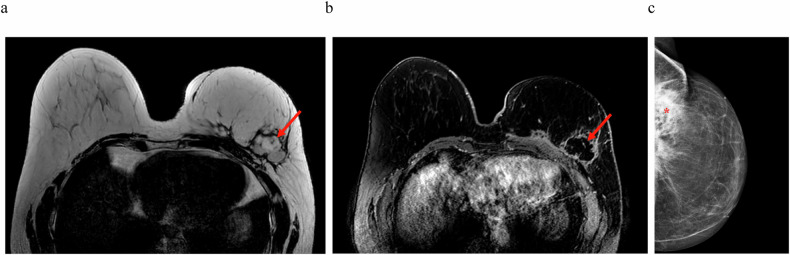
Fat necrosis after DIEP-flap breast reconstruction. A 67-year-old patient with a history of mastectomy because of DCIS underwent DIEP-flap reconstruction. Eight months after the reconstruction, fat necrosis was found in the lower outer quadrant of the left breast. MRI showed a lobed mass of 4.2 cm diameter, with surrounding tissue with mild enhancement and a specular aspect. The exam was classified BI-RADS 2 (a, b; arrow). Mammography showed coarse calcifications in the same area, probably related to the fat necrosis (c; asterisk)
